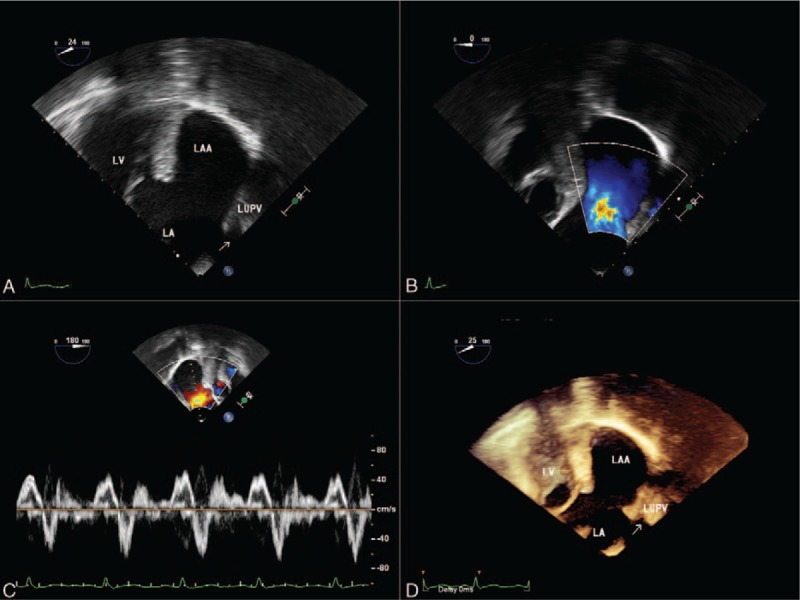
Transesophageal echocardiograms. The mid-esophageal 2-chamber and LAA view (A) revealing a giant LAA. Color Doppler flow imaging (B) showing communication of flow between LAA and the LA. Pulsed wave Doppler (C) echocardiographic examination of blood flow at the orifice. Three-dimensional view (D) confirming the presence of giant LAA. LA = left atrium, LAA = left atrial appendage, LV = left ventricle, LUPV = left upper pulmonary vein.
